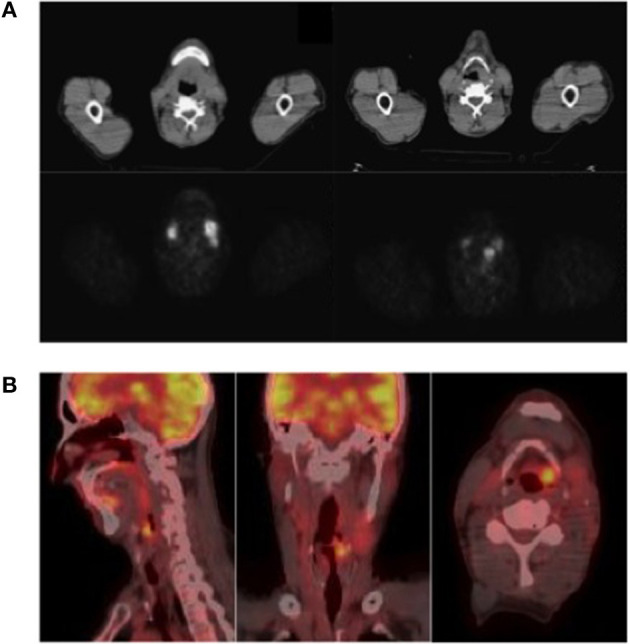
(A) 68Ga-DOTATOC PET-CT (upper panel) and (B) 18F-FDG PET-CT (lower panel). (A) 68Ga-DOTATOC PET-CT showed accumulation of radiotracer in left epiglottic region; (B) 18F-FDG PET-CT showed uptake of the radiotracer in the left emilarynx lesion, SUV max 9.3, and in two laterocervical lymph nodes, SUV max 11.4 and 7.
